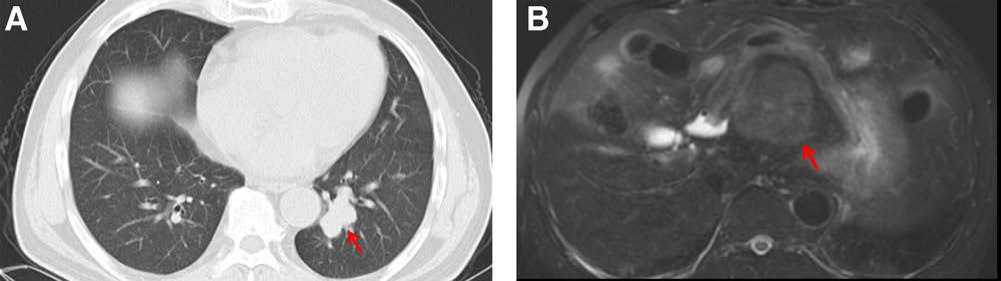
Baseline cross-sectional imaging (December 2019). (A) Chest CT showed a lobulated soft tissue nodule in the left lower lobe (red arrow; 3.1 cm × 2.3 cm). (B) MRI demonstrated a solitary hyperintense mass in the left hepatic lobe (red arrow; 5.9 cm × 4.7 cm). CT = computed tomography, MRI = magnetic resonance imaging.
